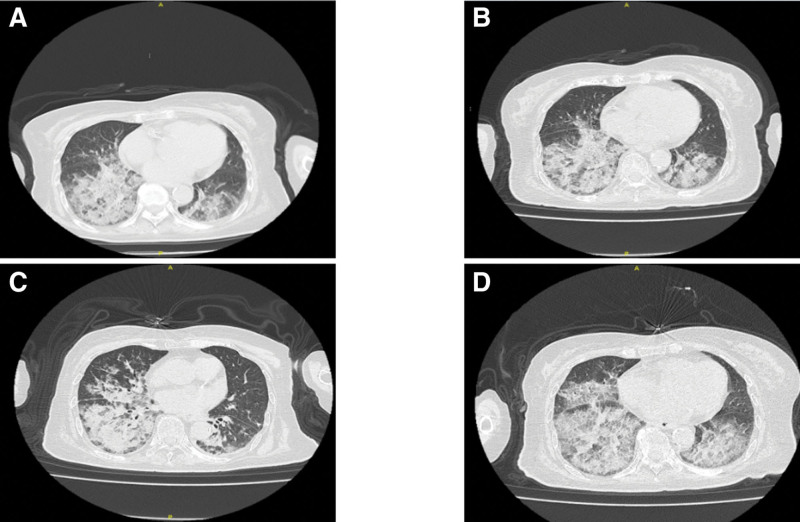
Chest computed tomography results of patients from May 2024 to March 2025: slow progression of pneumonia in patients. (A) May 27, 2024. (B) August 31, 2024. (C) November 10, 2024. (D) March 09, 2025.
